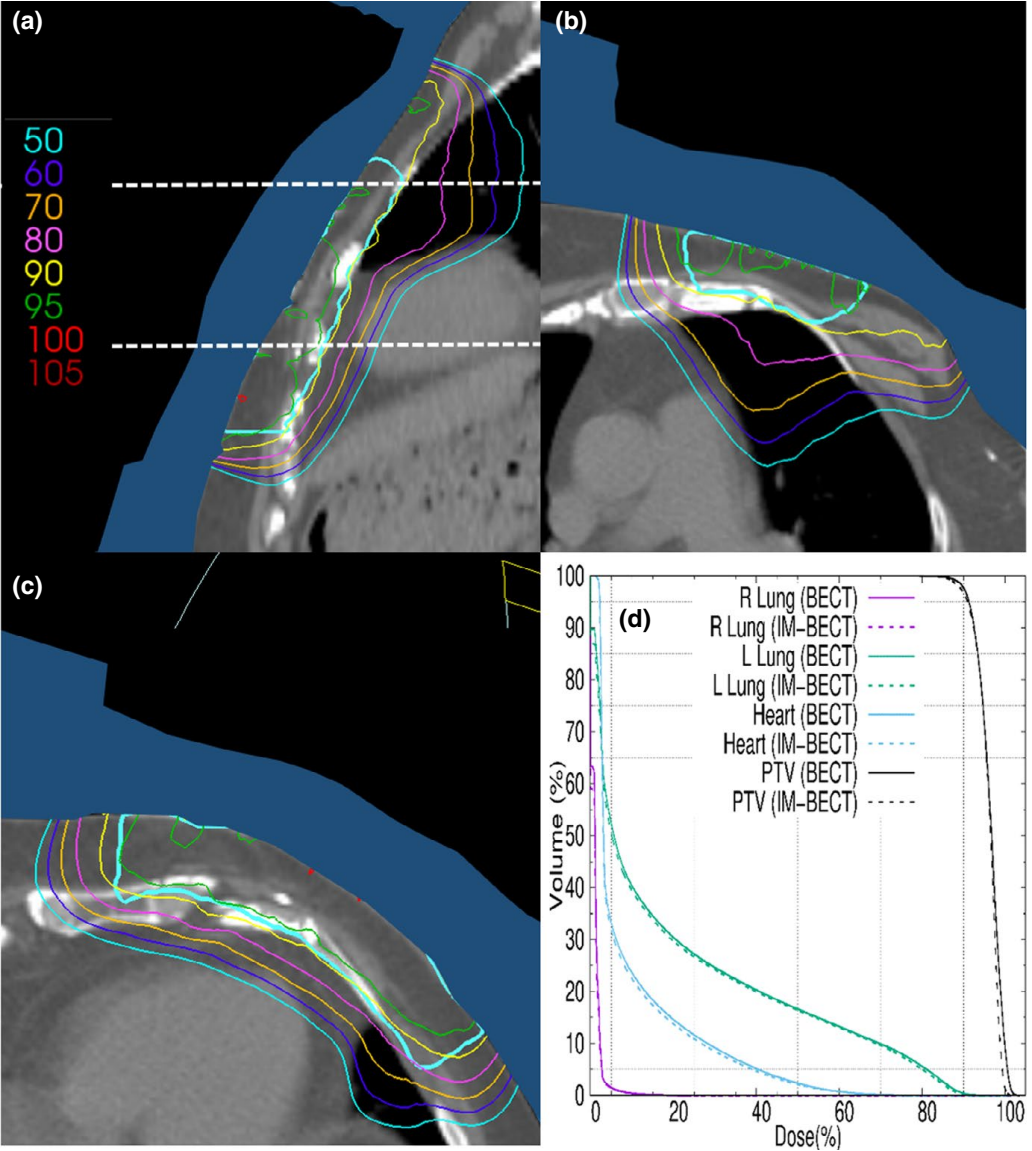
Chest wall patient CT planes showing the PTV (teal) and isodose lines (90% is yellow) for IM‐BECT treatment plan. The bolus is shaded blue. (a) Sagittal plane containing the central axis of the beam; dashed lines indicate positions of axial planes. Axial planes are (b) 3.5 cm superior (lung) and (c) 3.5 cm inferior (heart) to the central axis of the beam. (d) PTV, heart, right lung, and left lung DVHs for IM‐BECT and BECT treatment plans
